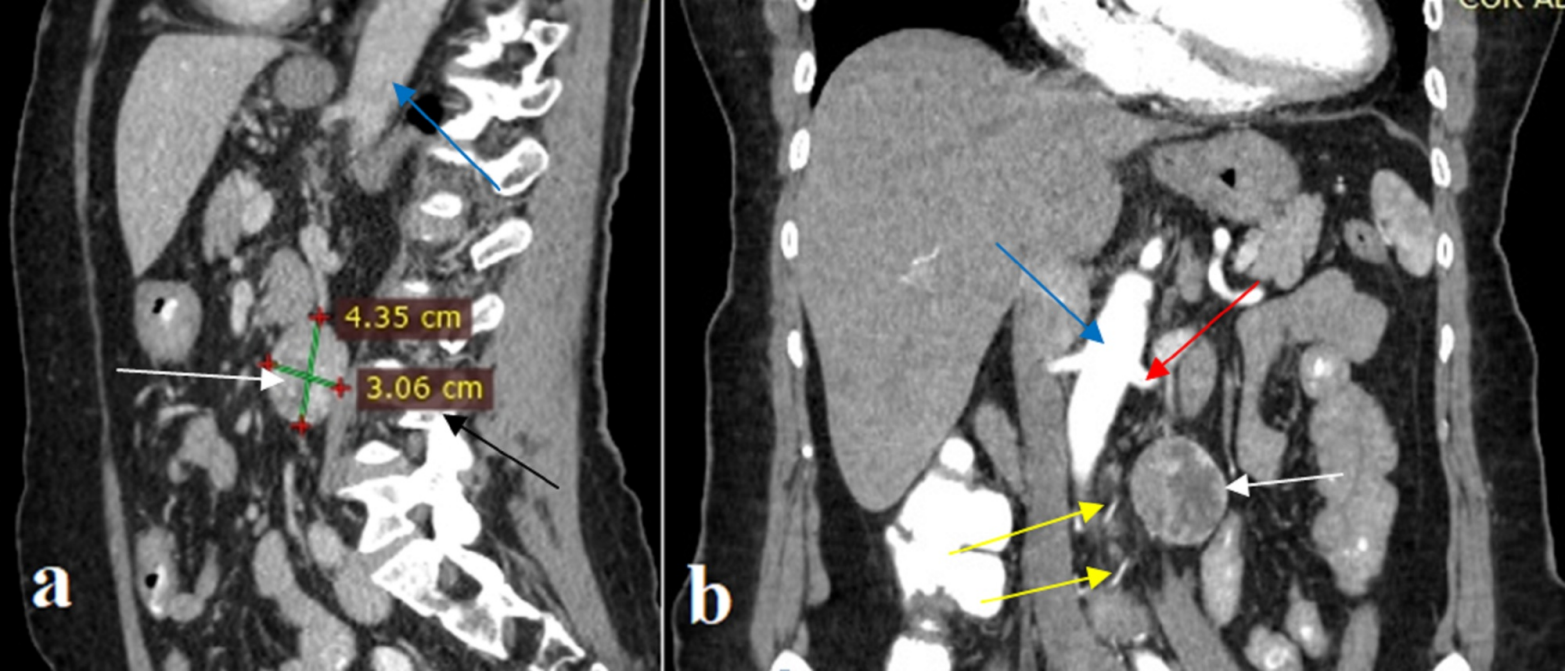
Preoperative abdominal CT scan a: sagittal view; b: coronal view. The images demonstrate a well-defined, left para-aortic, heterogeneous sizable mass (white arrow) located at the height of the fourth lumbar vertebra (black arrow), below the left renal artery (red arrow). Two arterial branches (yellow arrows) of the tumor’s vascular pedicle originating from the aorta (blue arrow) are recognized CT: computed tomography
